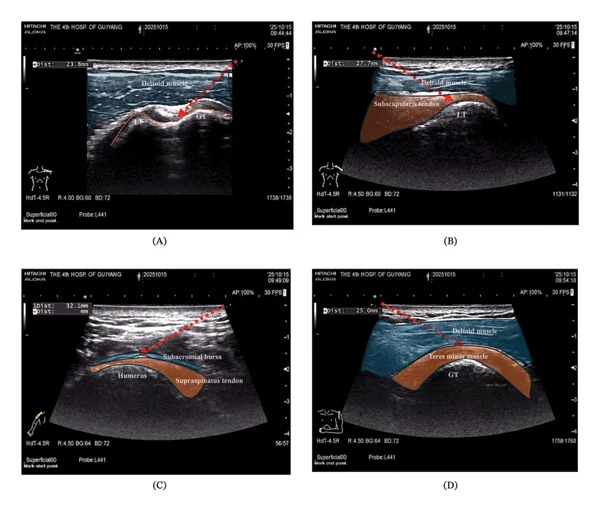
Ultrasound‐guided multitarget shoulder pericapsular injection pathway. (A) Anterior (subcoracoid/rotator‐interval) approach—advance the in‐plane needle to the anterior glenohumeral recess, carefully avoiding the long‐head biceps tendon. (B) Intertubercular groove, short‐axis view—insert the needle in‐plane from lateral to medial and deliver medication into the long‐head biceps tendon sheath (not intratendinous). (C) Posterior approach—in‐plane from superolateral to inferomedial, guide the needle into the posterior glenohumeral recess between the humeral head and posterior labrum. (D) Lateral coronal view—in‐plane from lateral to medial into the subacromial–subdeltoid bursa, creating visible bursal separation on injection. Abbreviations: LT, lesser tubercle; GT, greater tubercle.
